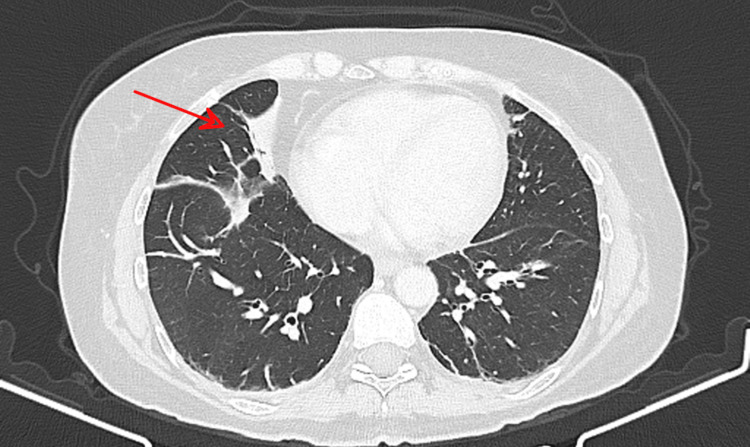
Chest CT 10 days after pembrolizumab administration Chest CT scan (lung window) shows an increase in band-like atelectasis involving the right middle lobe (red arrow). CT: computed tomography
